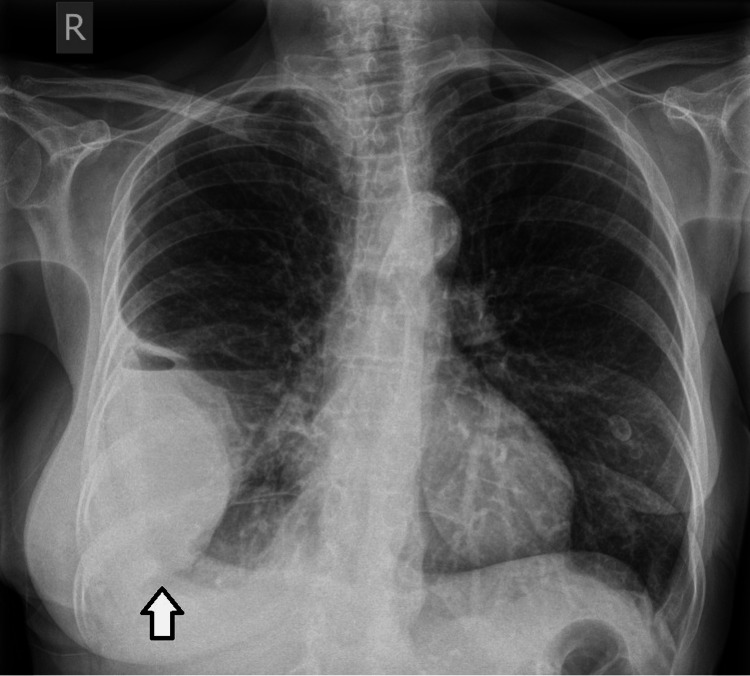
Chest X-ray on re-admission
